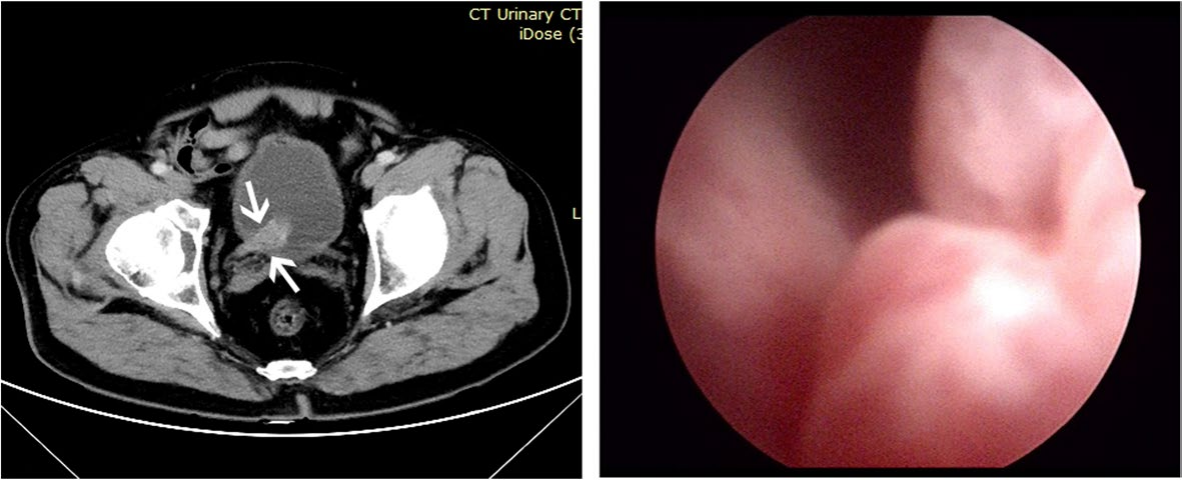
Representative views of the ureteral tumor by computed tomography-urography (CTU) and cystoscopy. a CTU showed an enlarged soft tissue shadow along the pelvic segment of the right ureter and continuous bladder trigone. b Cystoscopic examination revealed an irregular, polypoid, and pedicled lesion in the ureteral orifice
